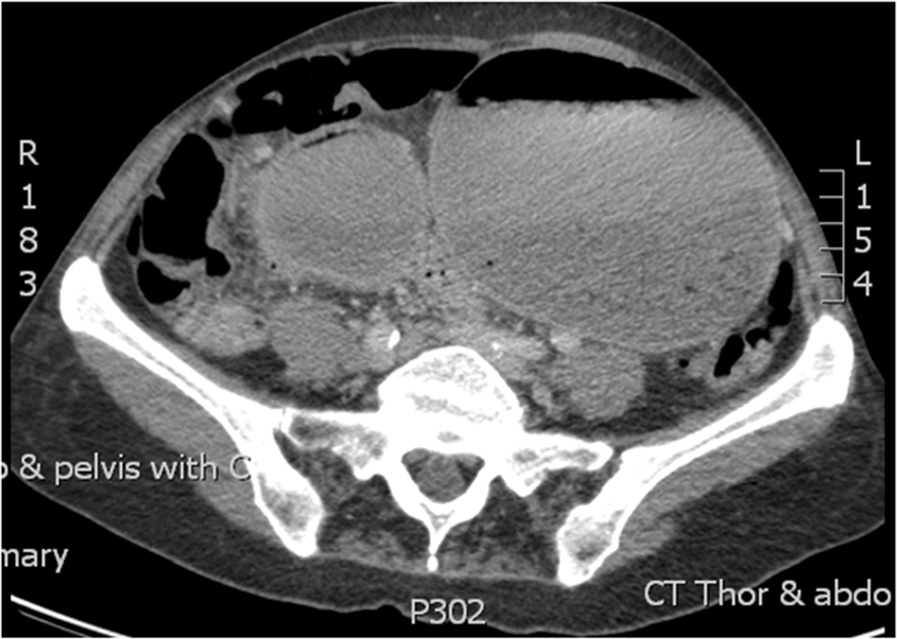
Computed tomography contrast image showing gastric outlet obstruction.
